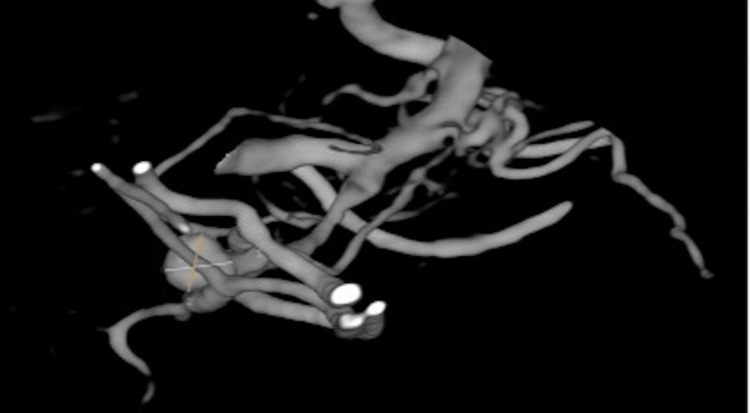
Computed tomography angiography showing an aneurysm measuring 4 x 4 mm
